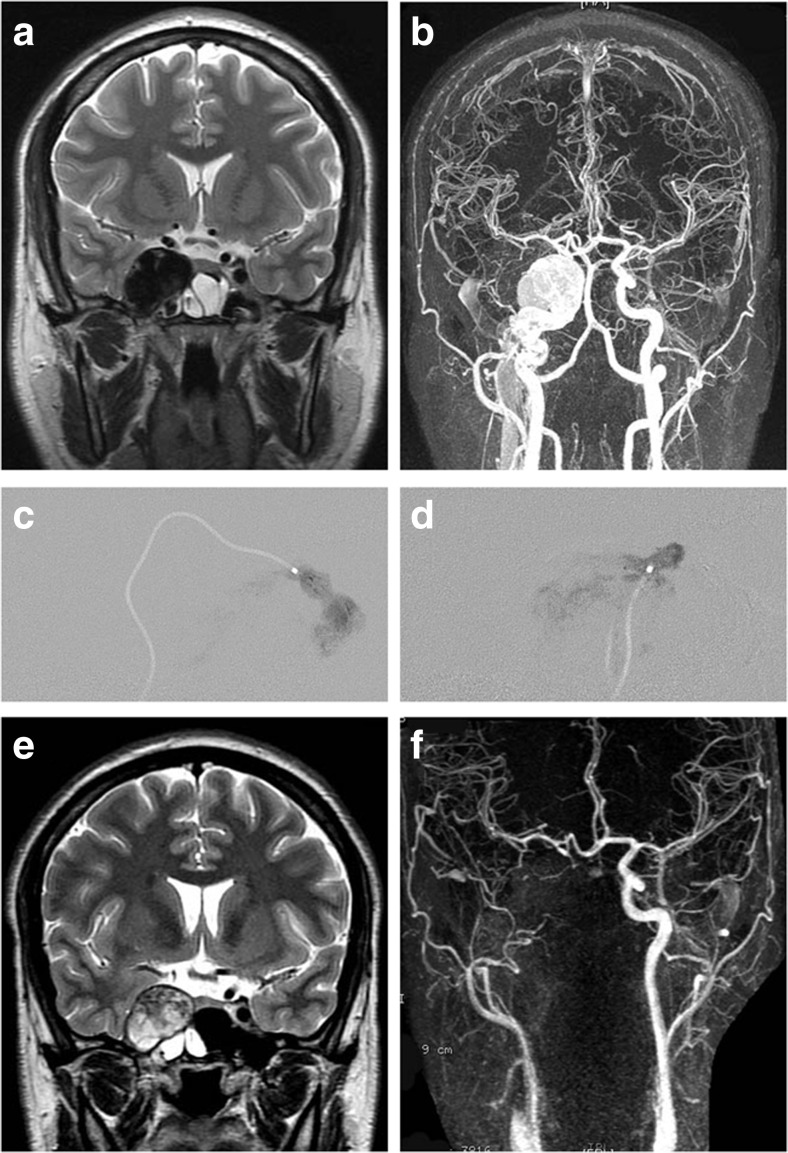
24-year old male patient with no clear head trauma recorded presented with a year-long history of pulsatile tinnitus and right sixth nerve palsy. a, b Preoperative MRI and MRA depicting right-sided CCF and a dilated cavernous sinus. DSA (not shown) excluded a ruptured intracavernous carotid aneurysm. It confirmed a CCF with a large tear in the ICA, draining posteriorly to the inferior petrosal sinus, with no direct supply to the ipsilateral hemisphere but adequate collateral circulation via anterior and posterior communicating arteries. Because of the suspicion of collagen disease and subsequent risks of balloon manipulation, it was decided to occlude the CCF by sacrificing the ICA. Glue injections were performed via the posterior communicating artery (c) and the intracavernous segment of the ICA (d), which sealed the CCF and occluded the ICA. e, f 1-month post-operative MRI and MRA demonstrating obliteration of the fistula and ICA. The patient reported full remission of symptoms. Note absence of MRI/MRA imaging artifacts after treatment with NBCA.
